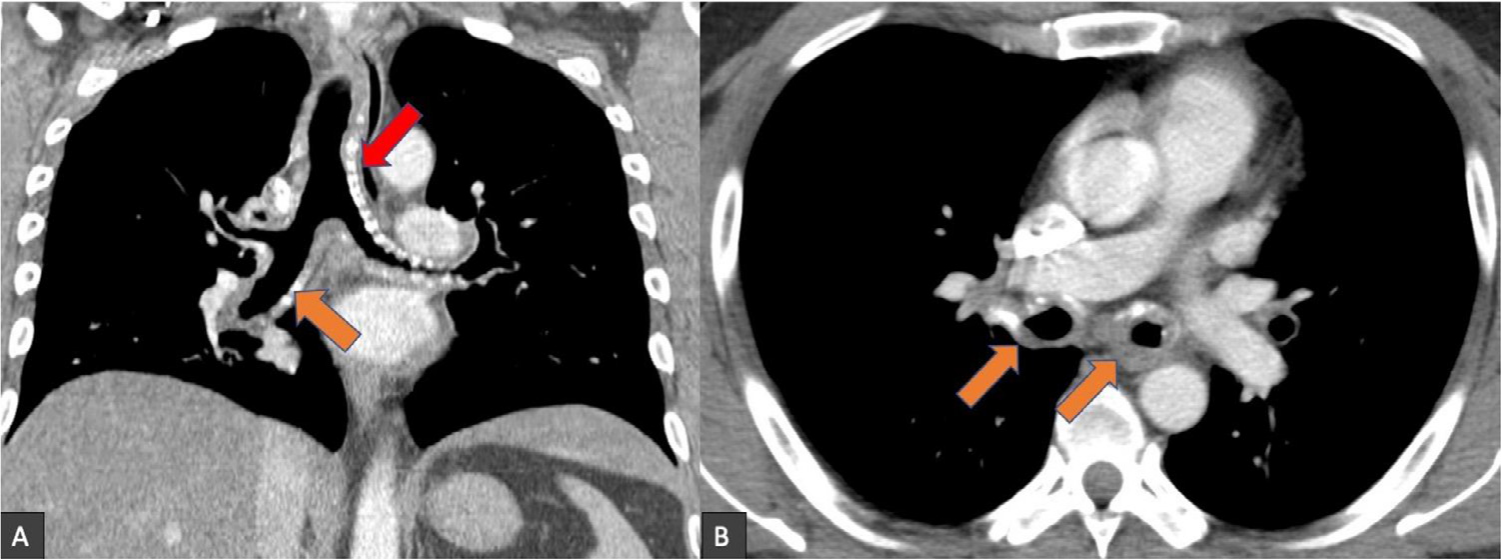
Coronal (A) and axial (B) contrast-enhanced CT of the chest demonstrates marked circumferential soft tissue thickening of the trachea (red arrow) and mainstem bronchi (orange arrows) with coarse calcifications, suggestive of tracheobronchial amyloidosis.
